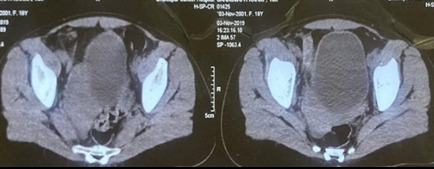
MRI scan after six cycles of NACT showed a well-defined heterogeneous density lesion with calcifications seen in the right peri vesical region, measuring approximately 5 x 1.7 cm.
